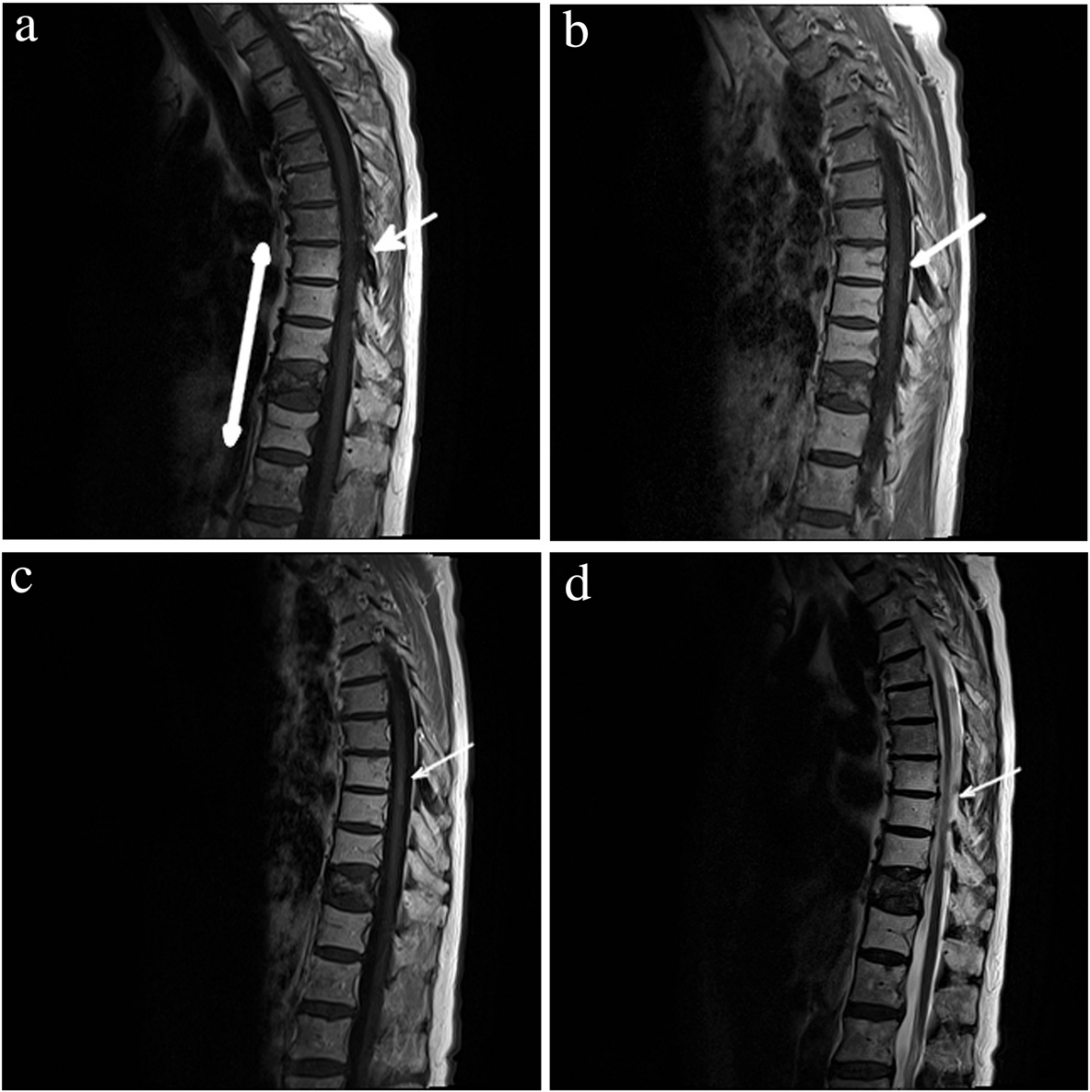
Thoracic cord magnetic resonance imaging of 71 year old patient in the course of radiation myelitis. a) February 2013: T1-weighted MRI scan without contrast shows hyperintense post-actinic changes in vertebrae Th 8-12, isointense changes in segments Th 7-9 and Th 11 fracture. b) February 2013: T1-weighted MRI with gadolinium demonstrates a ring-shaped posterior enhancement in spinal cord (arrow) at the level of Th 8-9. c) July 2013: T1-weighted MRI with gadolinium demonstrates a decrease of the ring-shaped posterior enhancement in spinal cord (arrow) at the level of Th 8-9 in comparison with the previous study (Figure 1b). d) July 2013: T2-weighted MRI scans demonstrate no signs of edema.
